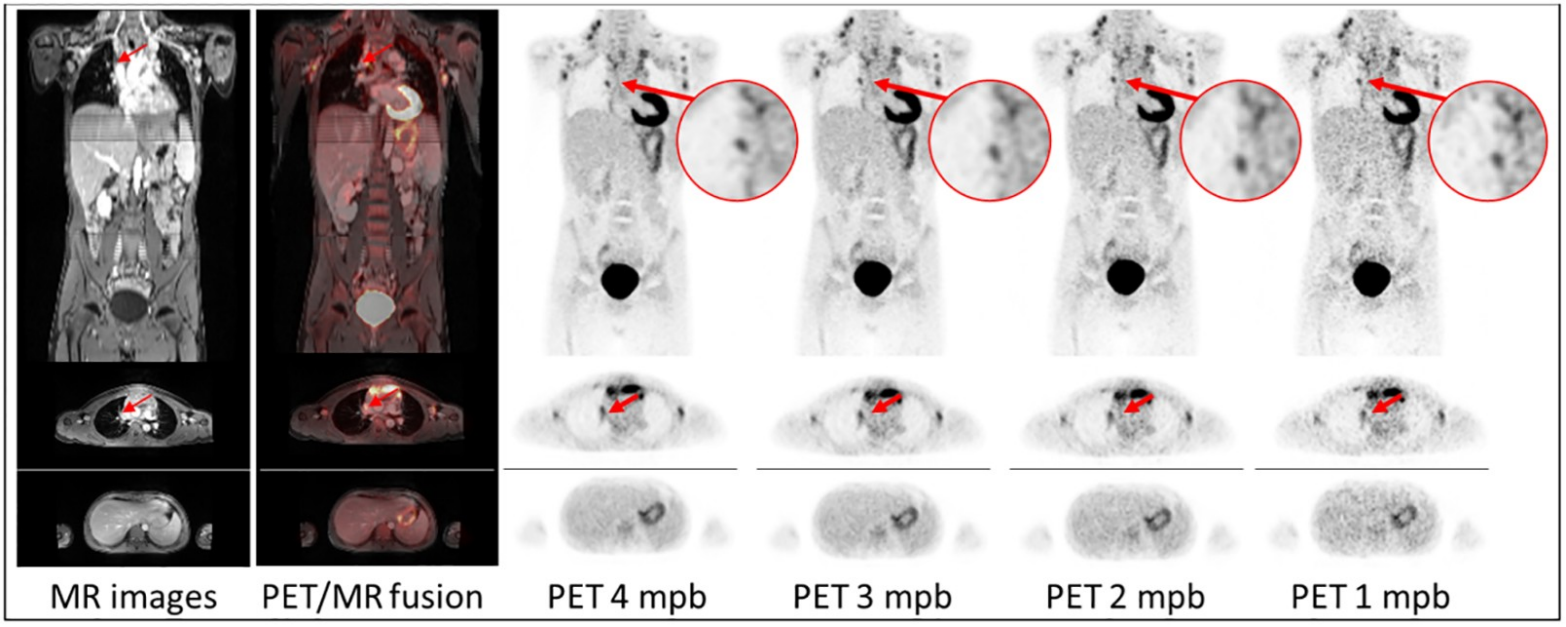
Patient example of consistently good image quality for different PET acquisition times. Example of consistently good image quality. MR images, PET/MR fusion images and PET images of patient #10 after 4, 3, 2 and 1 minutes per bed (mpb) position in coronal and axial orientation. The lesion is marked (red arrow). In addition an axial slice of the liver is shown. Discrete stripe artifacts in MR images result from coronal reformats at the positions where the transaxially acquired slice stack of two neighbored bed positions in the thorax are merged. Image noise (blood pool) increases from 18.7% at the 4 mpb to 23.7% in 1 mpb reconstruction. The CNR decreases from 28.3 at 4 mpb to 19.9 at 1 mpb. The SNR decreases from 14.8 at 4 mpb to 10.0 at 1 mpb.
